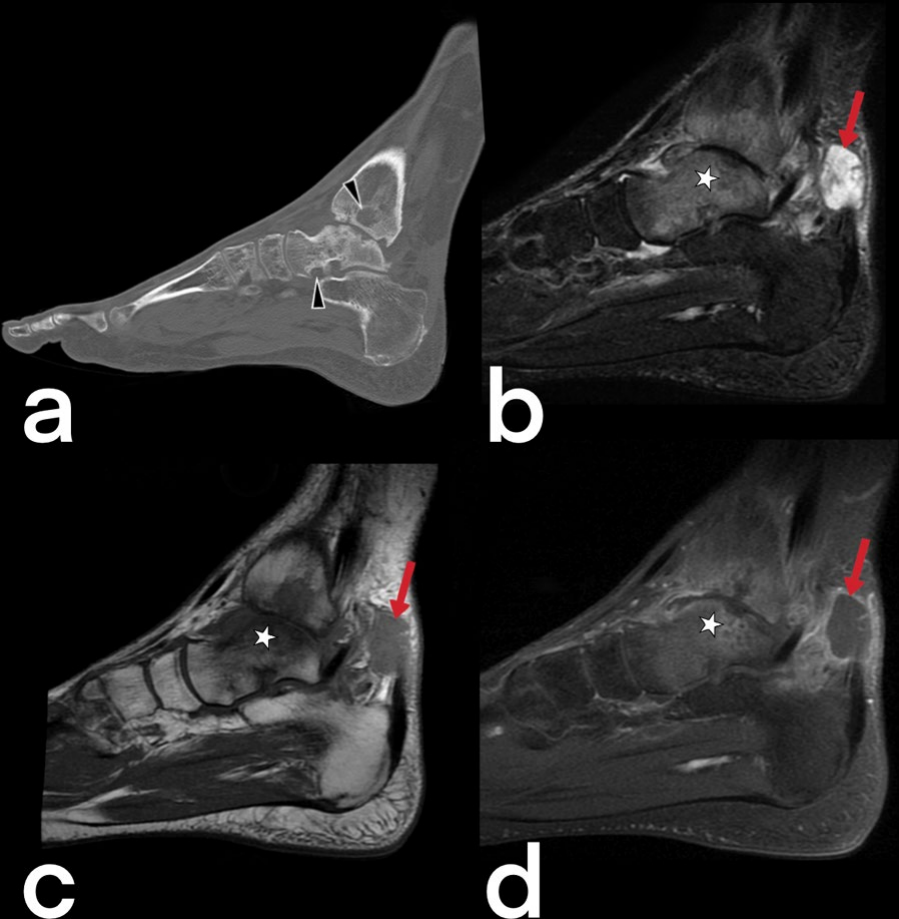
Sagittal reformatted CT (a) and MR images (b–d) of a 48-year-old male. CT image shows focal low-attenuation areas in the distal part of the tibia in keeping with abscesses and bone destructions (arrowheads). On sagittal T2-weighted (b), pre-contrast T1-weighted (c), and post-contrast T1 weighted (d) MR images reveal extensive signal intensity change within the bone marrow of the talus and distal tibial part (stars). MR images also demonstrate an abscess in the adjacent soft tissue to the Achilles tendon (arrows)
